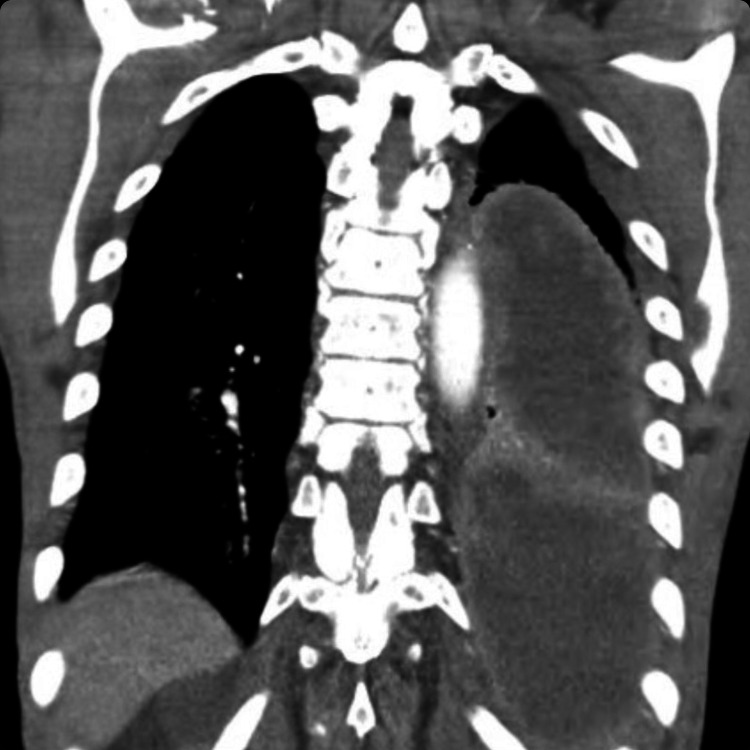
CT scan of the chest showing large left thoracic fluid collection (20 x 13 x 10 cm)
